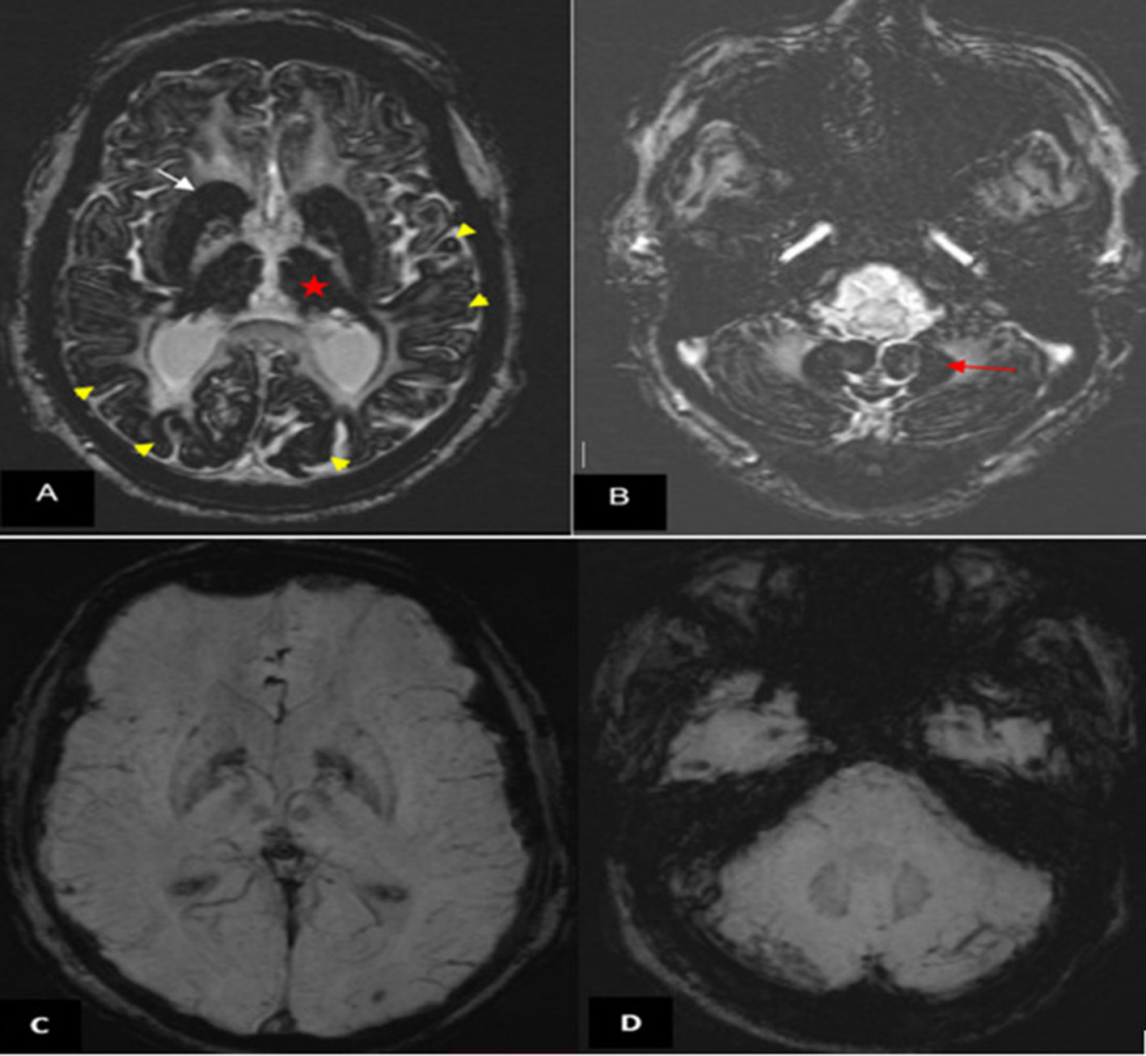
Brain MRI (1.5 T). (A, B) Axial SWI showing marked and extensive hypo intensity in the neo-striatum (white arrow), thalami (red star), cortex (yellow arrow head) (A) and dentate nuclei (red arrow (B)). (C, D) Normal SWI images in a matched patient followed for high blood pressure. SWI, susceptibility-weighted image.
